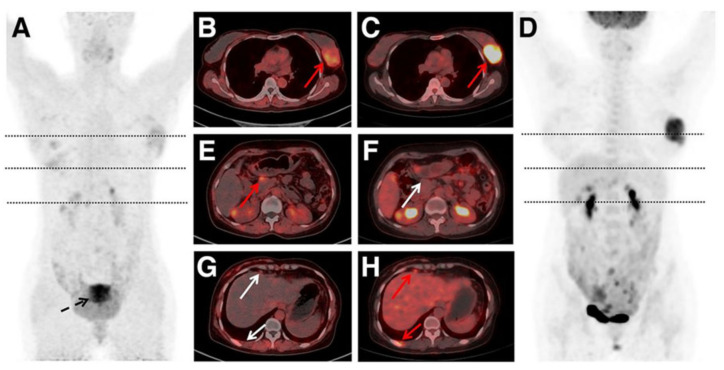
Images of ^68^Ga-FAPI and FDG PET/CT in a 46-year-old woman with lymphoblastic leukemia/lymphoma. (A) Maximum-intensity projection of ^68^Ga-FAPI PET. (B–H) Axial ^68^Ga-FAPI PET/CT images (B,E,G) and ^18^F-FDG PET/CT images (C,F,H). (D) Maximum-intensity projection of FDG PET. Left breast involvement was detected with intense ^68^Ga-FAPI and FDG uptake (B,C, arrows). PET/CT image showed lesion with focal ^68^Ga-FAPI uptake in pancreas (E, arrow) and normal FDG activity (F, arrow). FDG PET/CT image showed lymph nodes positive for uptake in internal mammary and subpleural areas (H, arrows), without corresponding ^68^Ga-FAPI uptake (G, arrows). Intense ^68^Ga-FAPI uptake was noted in uterus (A, arrow). (Figure from [87]. Copyright © Society of Nuclear Medicine and Molecular Imaging).
